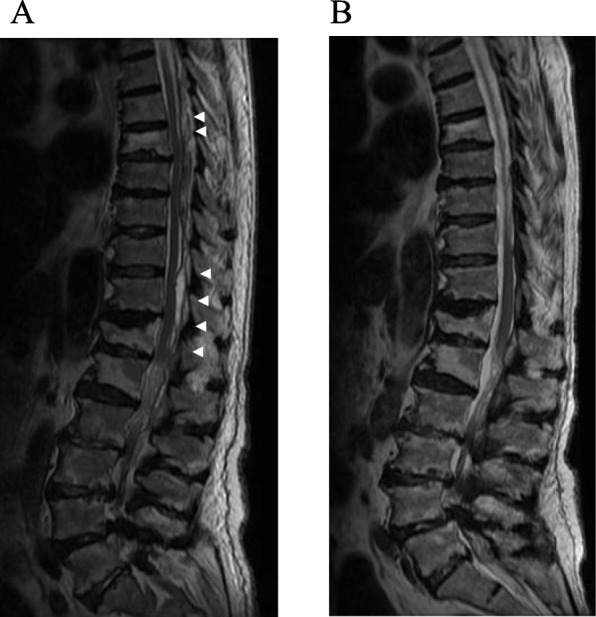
a Magnetic resonance imaging (MRI; T2) performed a day after fluoroscopy-guided percutaneous puncture. The volume of fluid in the epidural space has decreased and the spinal cord compression has been relieved (arrow). b MRI (T2) performed after 3 weeks. The epidural fluid pool has completely disappeared
